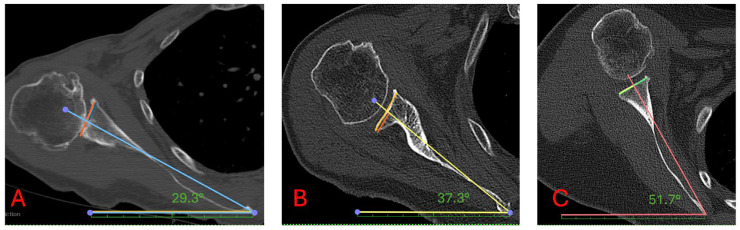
Measurement of the Scapular Internal Rotation (SIR) on axial CT reconstructions. Colored lines in each plane depict the anatomical landmarks and reference axes used to compute the inclination angle [20]. Image (A) shows an angle of 29.3°, image (B) shows an angle of 37.3°, and image (C) shows an angle of 51.7°.
